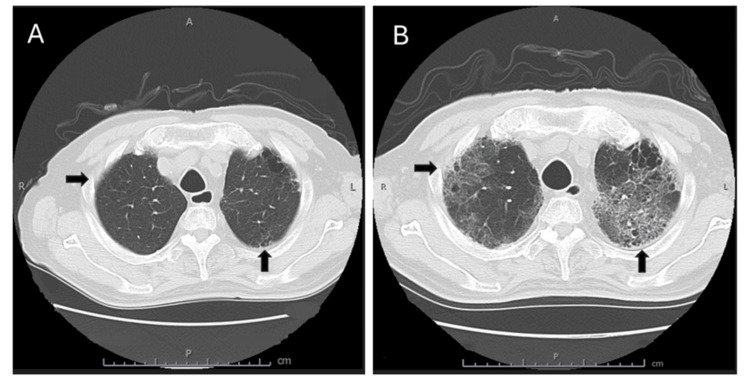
CT images of this case taken before starting apalutamide treatment and at the time of hospitalization (day 65). CT: Computed tomography A. CT images taken before starting apalutamide treatment did not show NSIP. B. CT images taken at the time of admission (day 65) suggested NSIP.
